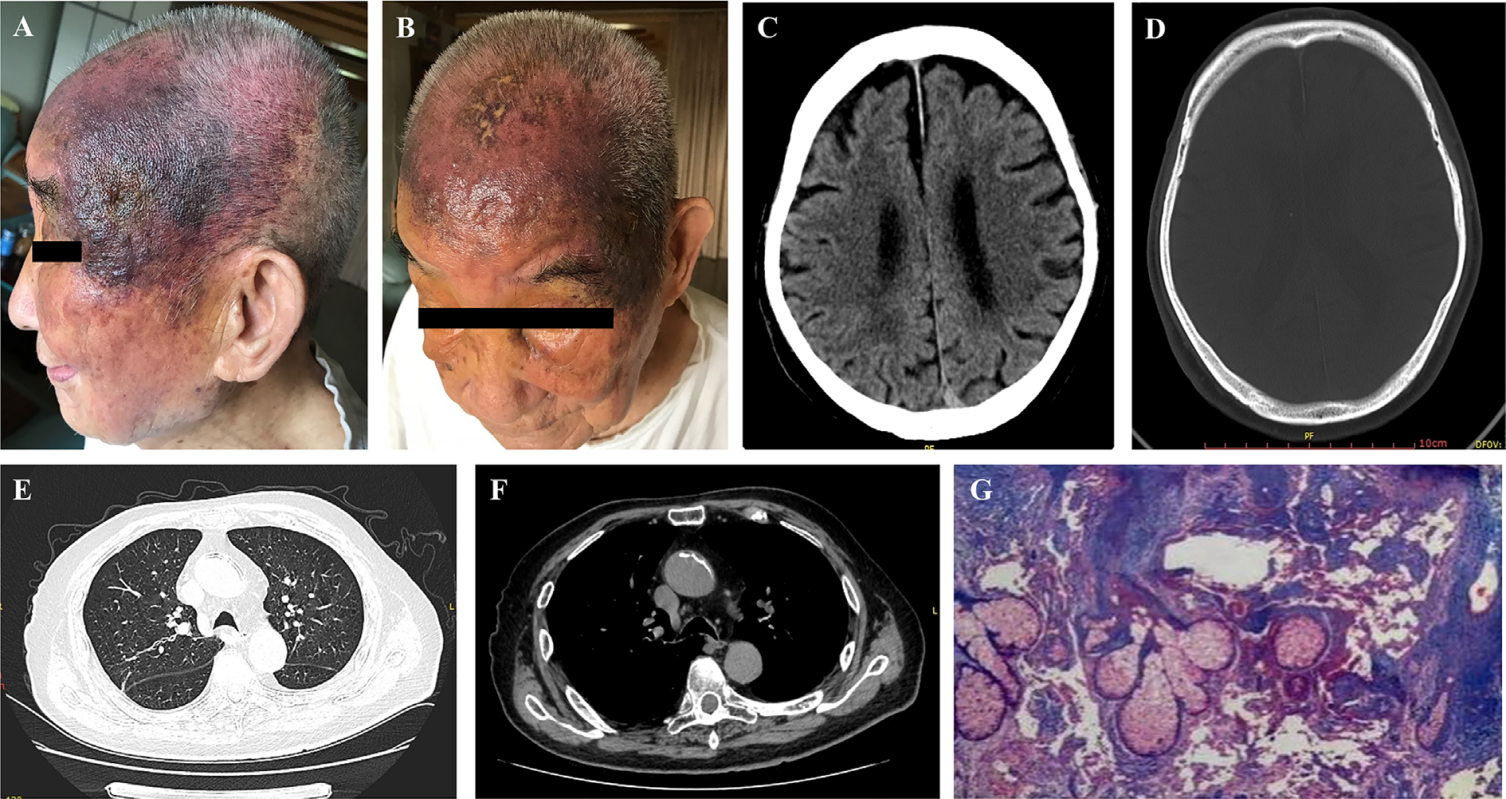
Examination before treatment including dermatological inspection (A, B), head CT (C, D), chest CT (E, F) and pathological assessment (G).
